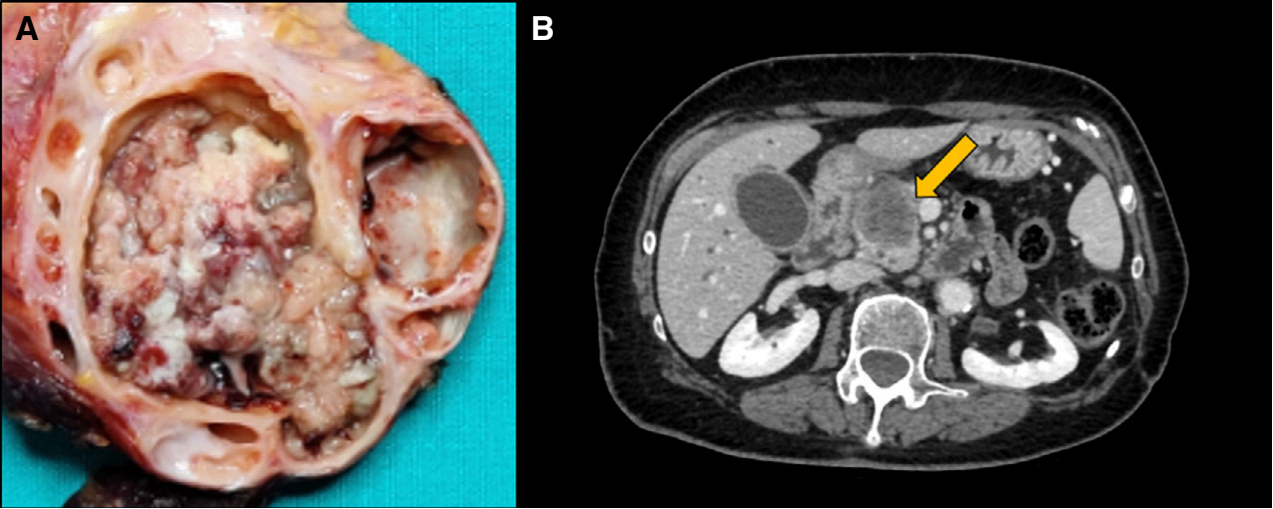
Typical appearance of a case of pancreatic ITPN, showing solid and solid/cystic areas (A: solid/cystic appearance at gross sampling, B: solid and solid/cystic appearance at imaging: CT scan, where the lesion is indicated by a yellow arrow). [Colour figure can be viewed at wileyonlinelibrary.com]
